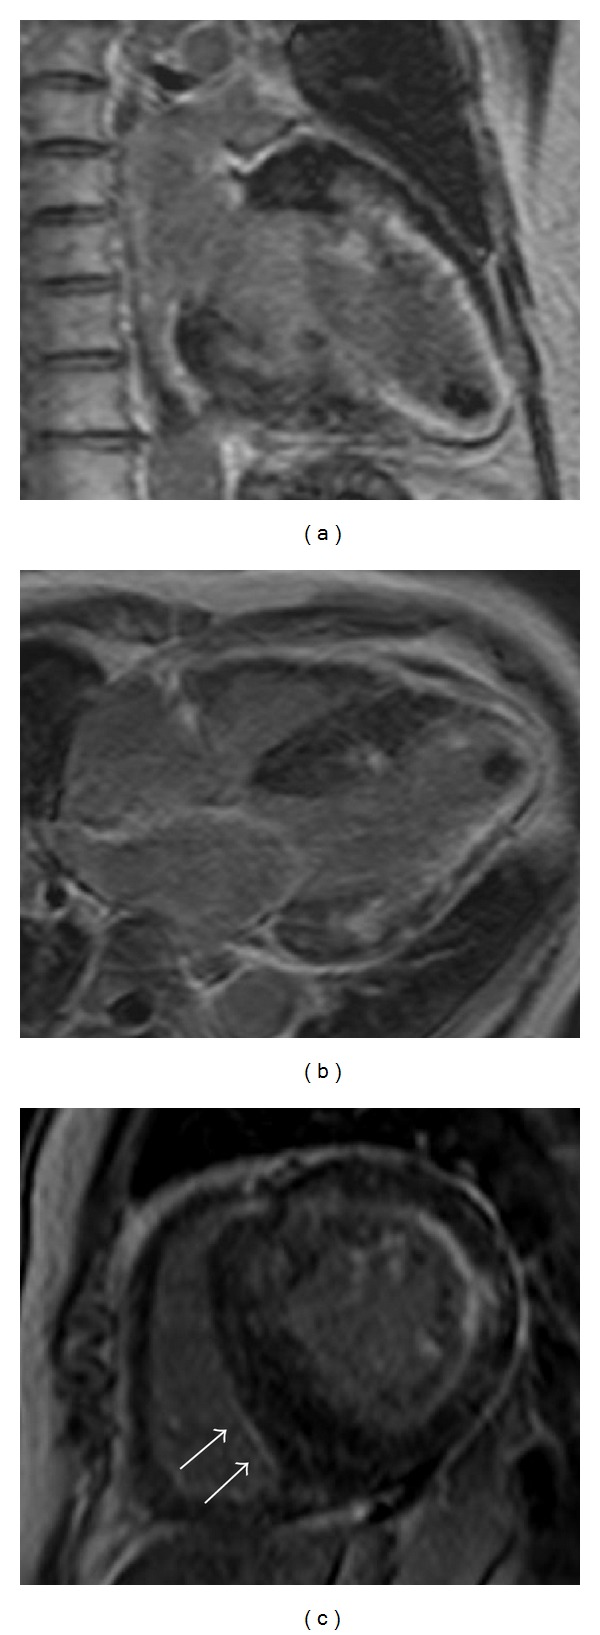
The long-axis view (a), four-chamber view (b), and short axis view (c) of cardiac magnetic resonance imaging revealed a thin-walled apical aneurysm, with subendocardial late gadolinium enhancement (LGE) in an extensive area of the mid-to-apex region of the left ventricle and in a smaller area of the right ventricle (small arrows). LGE involved the majority of the basal lateral wall. Smaller midmyocardial LGE located in the basal interventricular septum, the wall of which was markedly thickened, was also observed. Scanner type: 3D-FFE, matrix scan: 224, flip angle: 15 degrees, and bandwidth: 324.7 Hz. The repetition time, echo time, and inversion time were (a) 3.910, 1.219, and 280 m/s, (b) 4.005, 1.250, and 290 m/s, and (c) 4.078, 1.272, and 300 m/s, respectively.
